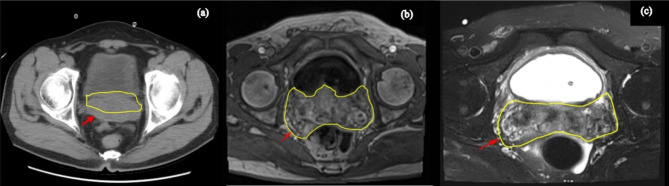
(a) Pelvic CT scan shows an obvious enlargement of the seminal vesical (b) Pelvic MRI T1 and (c) Pelvic MRI T2 suggest that the arrows point to a region of heterogeneous signal in the seminal vesicle
